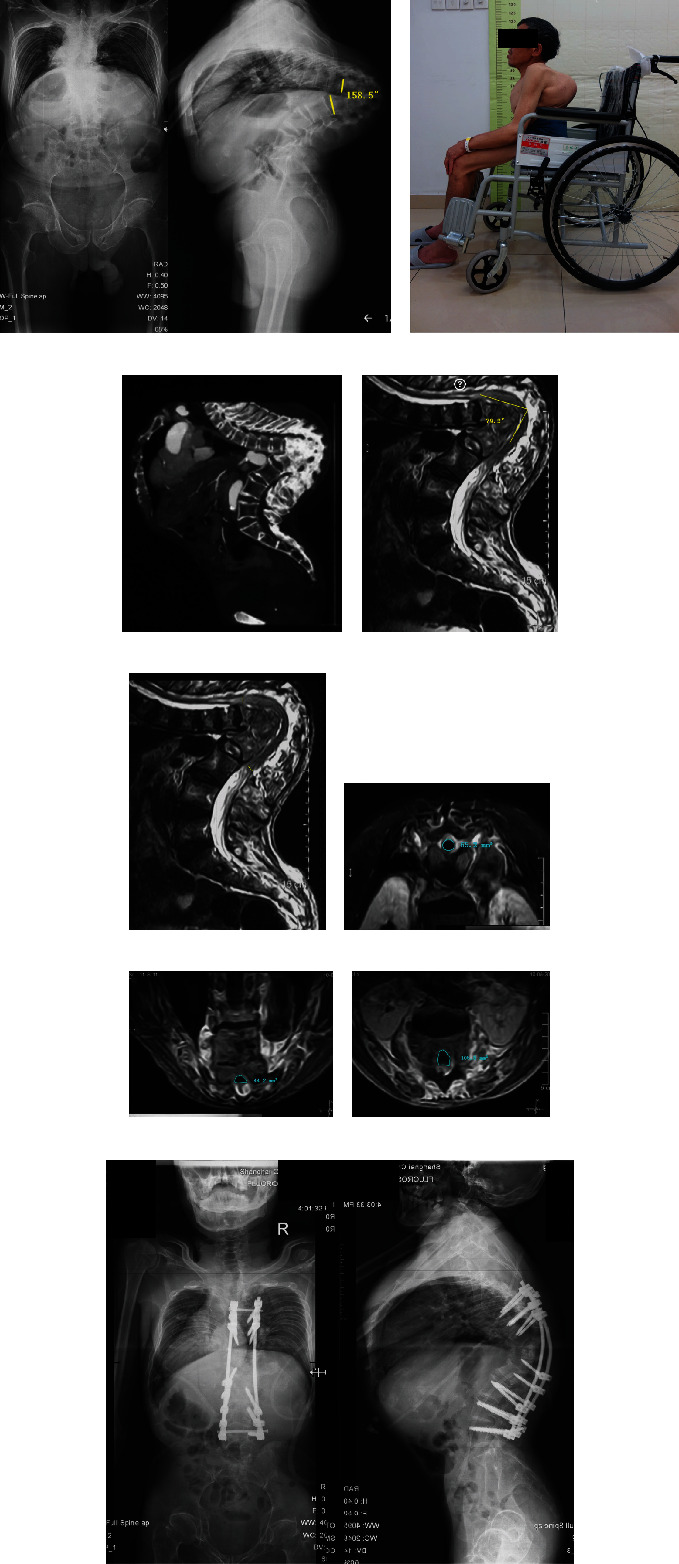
A 63-year-old male with spinal tuberculosis presented with 158.5° of kyphosis (a). (b) Preoperative photograph showed obvious stoop (AIS grade B). The patients suffered from delayed paraplegia. (c) CT revealed destruction and fusion of multiple vertebrae from T7 to L1. (d) T2-weighted MRI showed compressed spinal cord in the kyphotic region. SCA was 79.2°. (e) SDR was 64.3%. (f–h) CSAR was 48.3%. (i) MVCR surgical procedure was carried out to resect the vertebrae of T8-12, with posterior pedicle screw fixation and fusion. SDR: spinal cord sagittal diameter ratio; SCA: spinal cord angle; CSAR: spinal cord cross-sectional area ratio.
